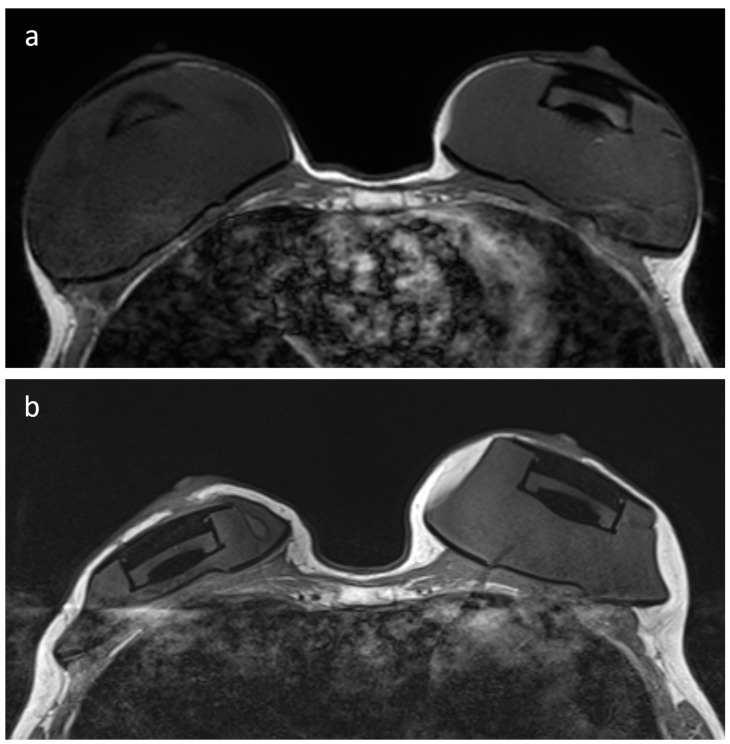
(a) T1-weighted Dixon in-phase image of a patient that underwent bilateral nipple sparing mastectomy and pre-pectoral implantation of MRI-conditional breast tissue expanders, showing two intact expanders in place. (b) MRI examination showing partial deflation of the right tissue expander after iatrogenic rupture. Even after substantial deflation of the tissue expander, the RFID-related artifact of the expander port does not impair the visualization of surrounding breast tissue, skin, and chest wall structures.
